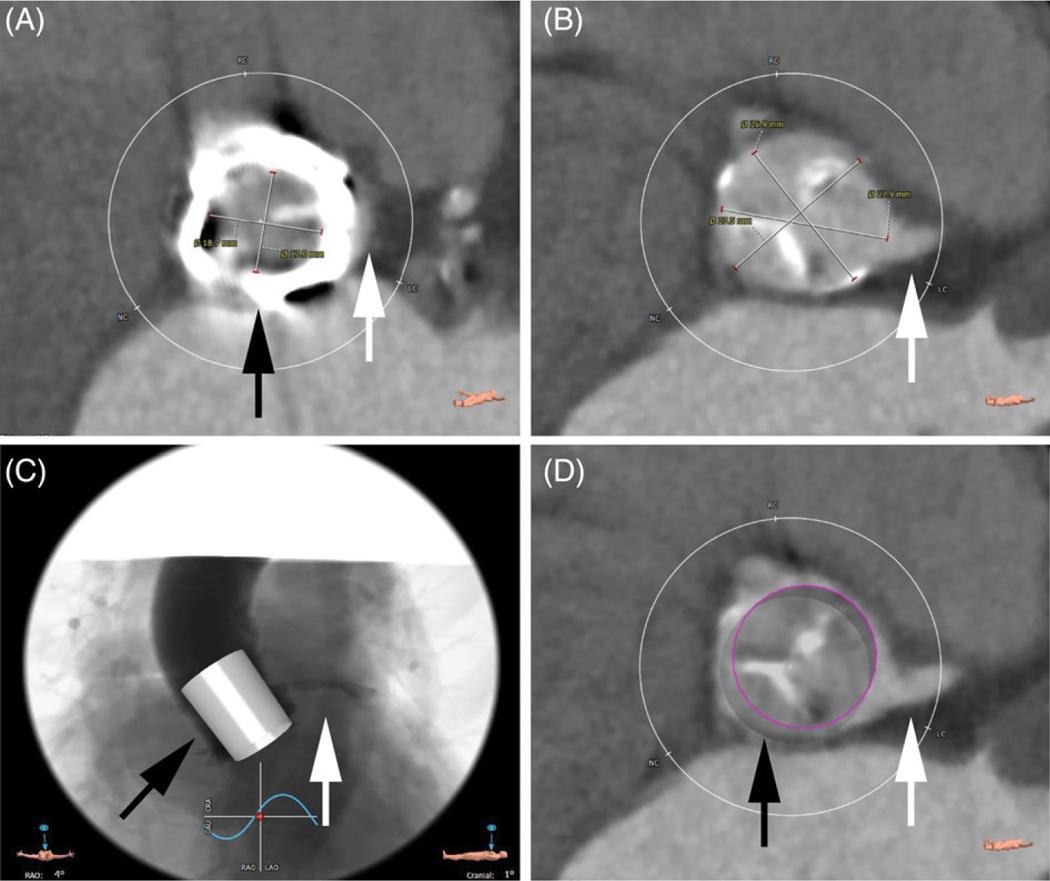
Preoperative CT modeling for case 1. A, Surgical valve frame (black
